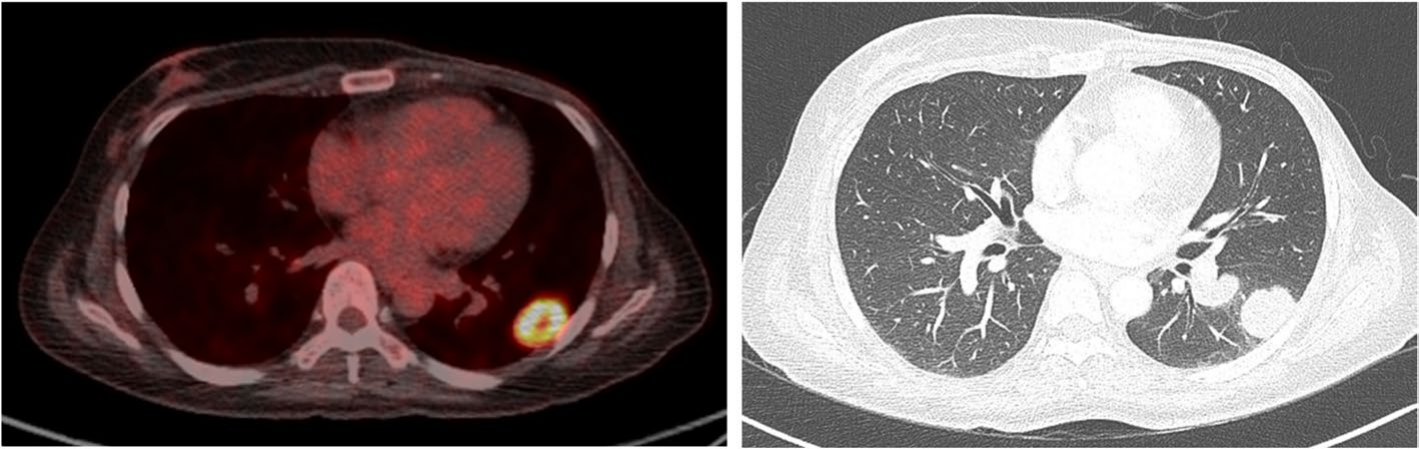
Both PET and chest CT showed multiple metastases in the left lung at one and a half years after surgery
